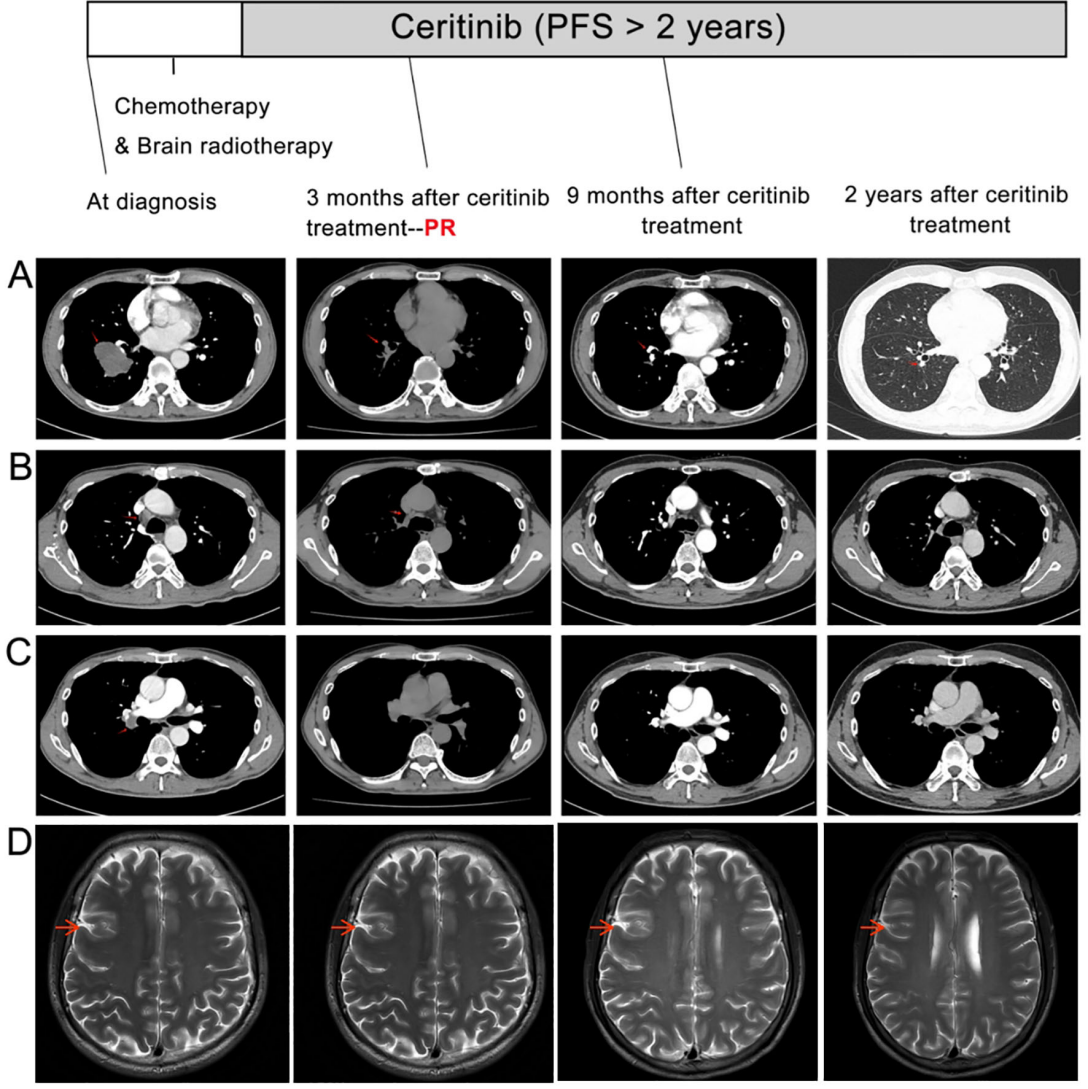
Treatment response on imaging. (A-C): Baseline chest computed tomography (CT) scans at diagnosis showing (A) a 4.8 cm (longest diameter) ×3.5 cm mass in the right lower lobe (white arrow), (B) obstructive pneumonia, and (C) enlarged hilar/mediastinal lymph nodes (arrowheads). (D): Baseline brain magnetic resonance imaging (MRI) revealing a metastatic lesion in the right frontal lobe (red arrow). Lower panels: Follow-up imaging at 3, 9, and 24 months after initiation of ceritinib (note: one cycle of platinum-based chemotherapy and whole-brain radiotherapy were administered between baseline imaging and the start of ceritinib) demonstrating (A–C) significant regression of the primary mass (2.4 cm × 1.2 cm at 3 months, yellow arrow), resolution of obstructive pneumonia, and reduced lymphadenopathy; and (D) complete resolution of the brain metastasis. RECIST assessment: The primary target lesion’s longest diameter decreased by 50% (from 4.8 cm to 2.4 cm), meeting partial response (PR) by RECIST 1.1 criteria (≥30% decrease in the sum of longest diameters of target lesions). The intracranial lesion met criteria for complete response on MRI.
